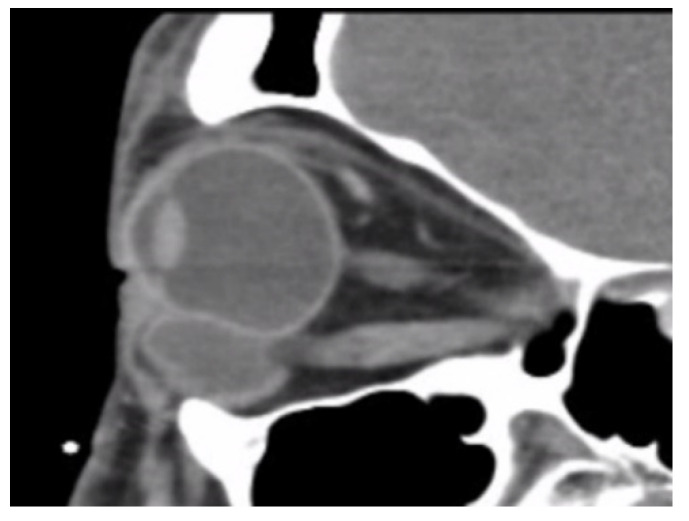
Orbital CT showed anteriorly located hydrogel scleral buckles.
